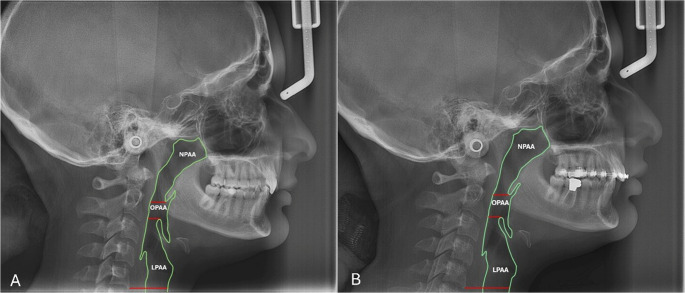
SPAD changes at the three tested levels; NPAA, OPAA, and LPAA, as measured from lateral cephalometric x-rays. (A) Pre-treatment at T0, and (B) Post-treatment at T1
